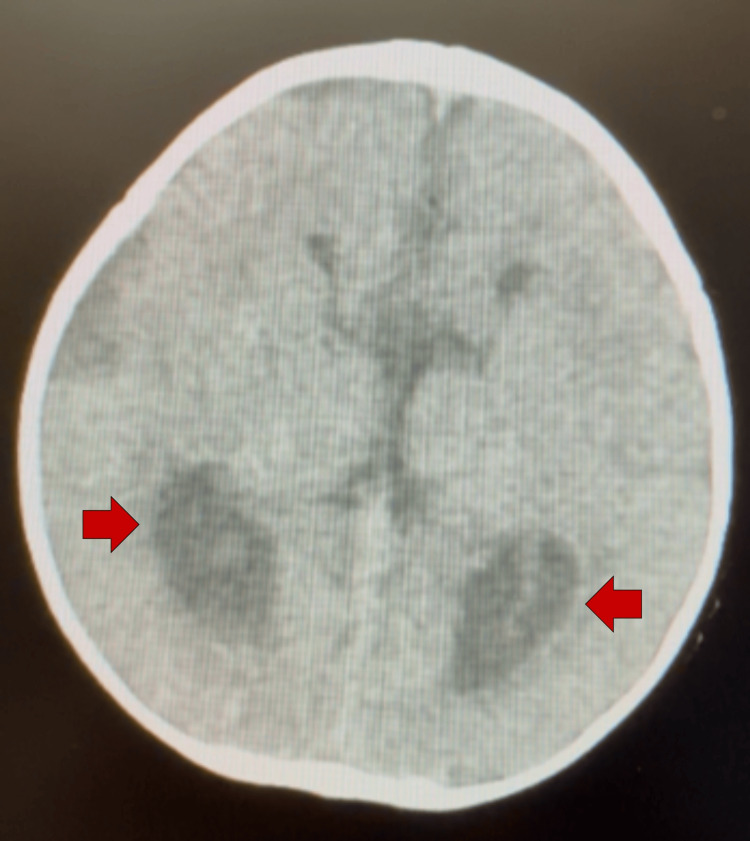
Axial CT of the brain Axial CT of the brain demonstrates widely spaced lateral ventricles with prominent dilation of the occipital horns, identifying colpocephaly (red arrows). This ventricular displacement creates a “race car” configuration characteristic of agenesis of the corpus callosum.
